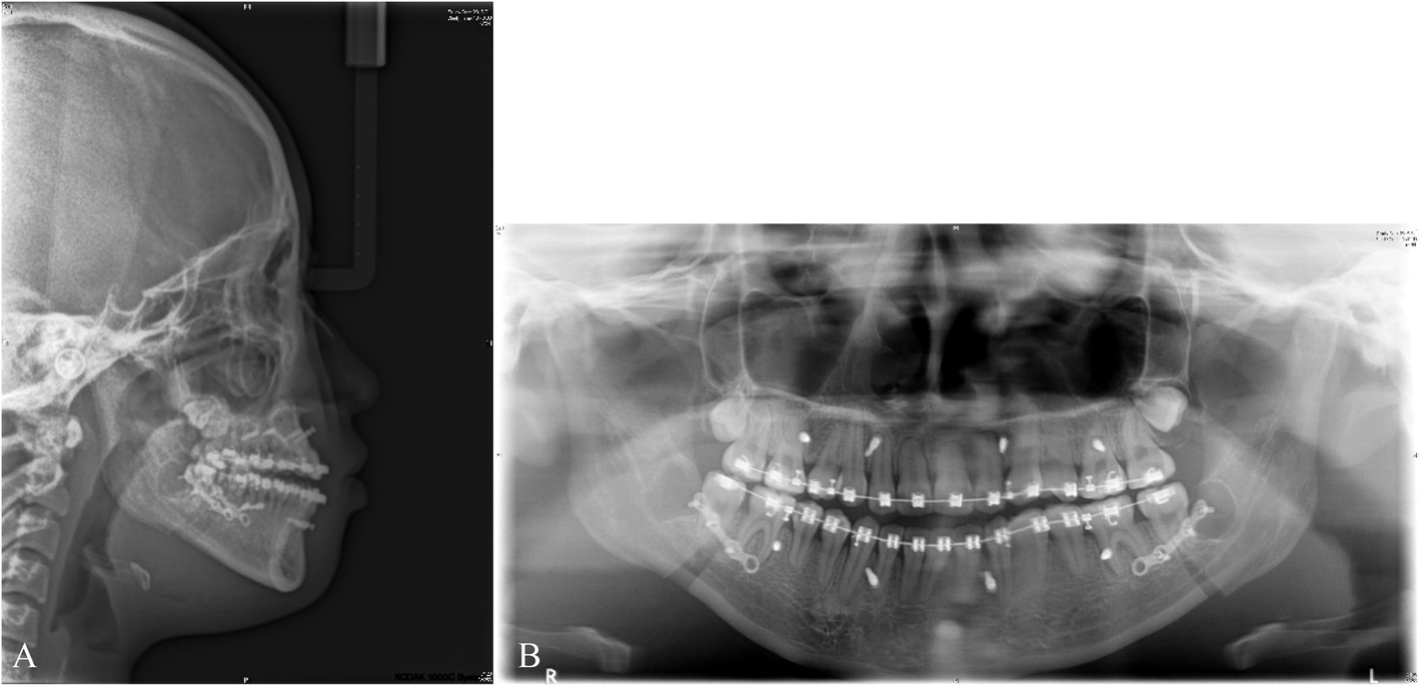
A, B Lateral cephalometric and panoramic radiographs 2 weeks after BSSRO of #1. 26 months after the MASDO procedure, BSSRO was performed to correct the patient’s mandibular prognathism and anterior crossbite. The amount of mandibular setback was approximately 4.0 mm on both sides
